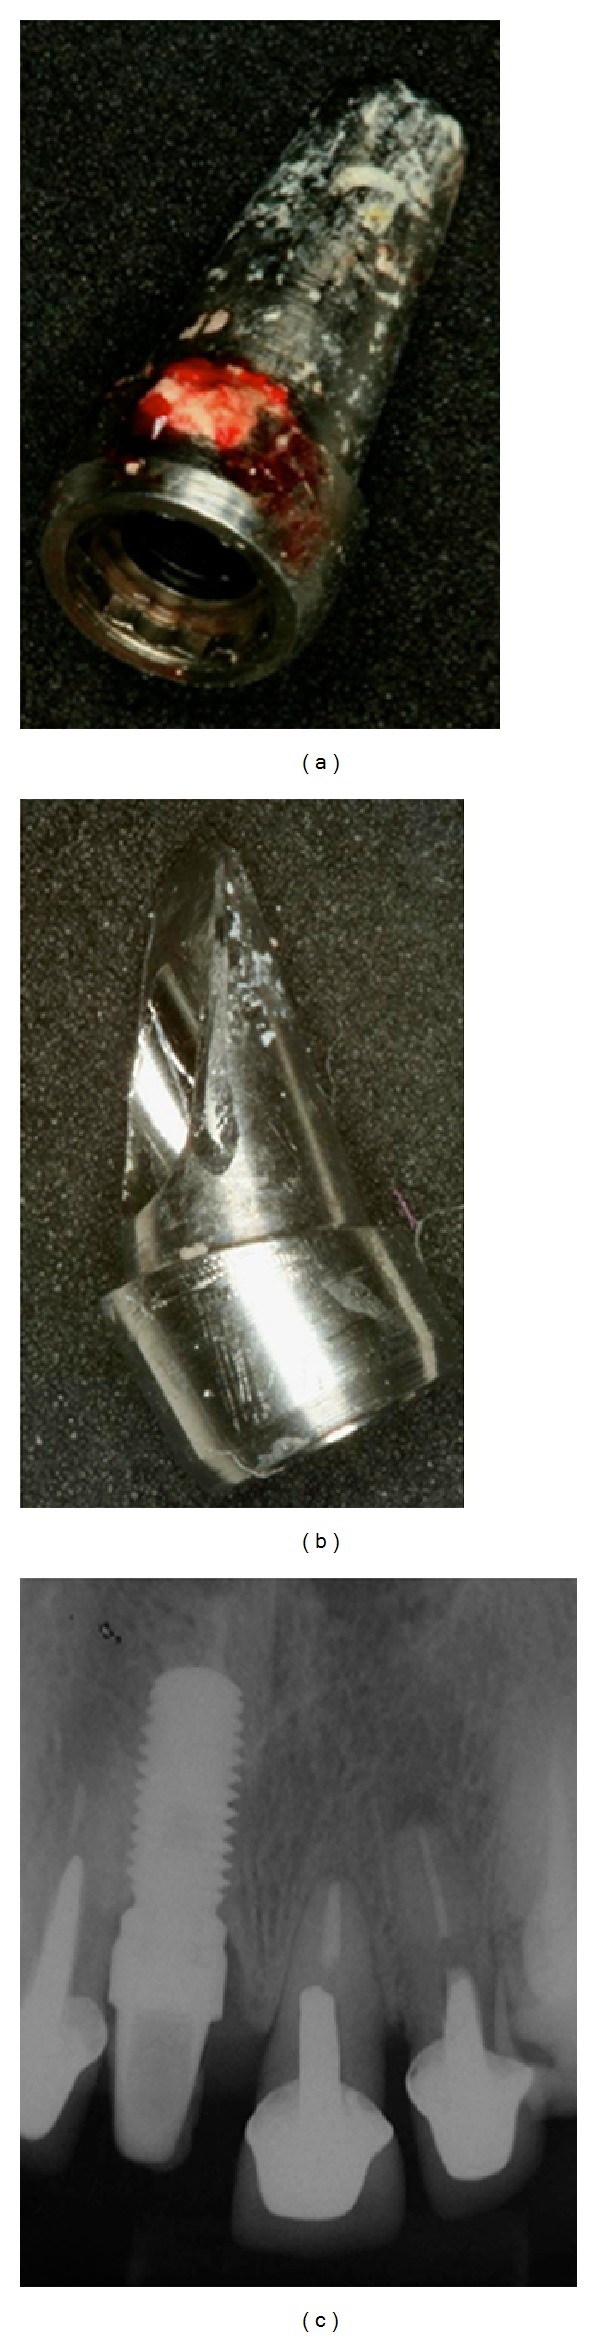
(a) Right maxillary central incisor abutment removed. (b) Angled abutment to correct the implant malpositioning; (c) periapical radiograph revealed endodontic treatment of the left maxillary central incisor and the left maxillary lateral incisor and the root resorption of left maxillary lateral incisor.
